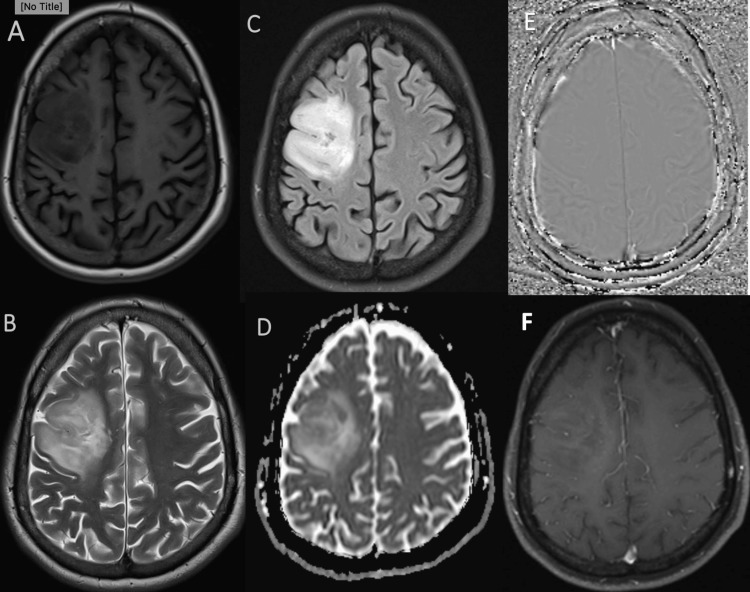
MRI of right frontal lobe diffuse astrocytoma showing hypointense signal on T1WI (A), hyperintensity seen on T2WI/FLAIR image (B, C). No diffusion restriction on the ADC image (D), no calcification/blood products in the filtered phase image (E), and no enhancement in the post-contrast image (F). ADC: Apparent diffusion coefficient, FLAIR: Fluid attenuated inversion recovery, T1WI: T1 weighted image, T2WI: T2 weighted image.
